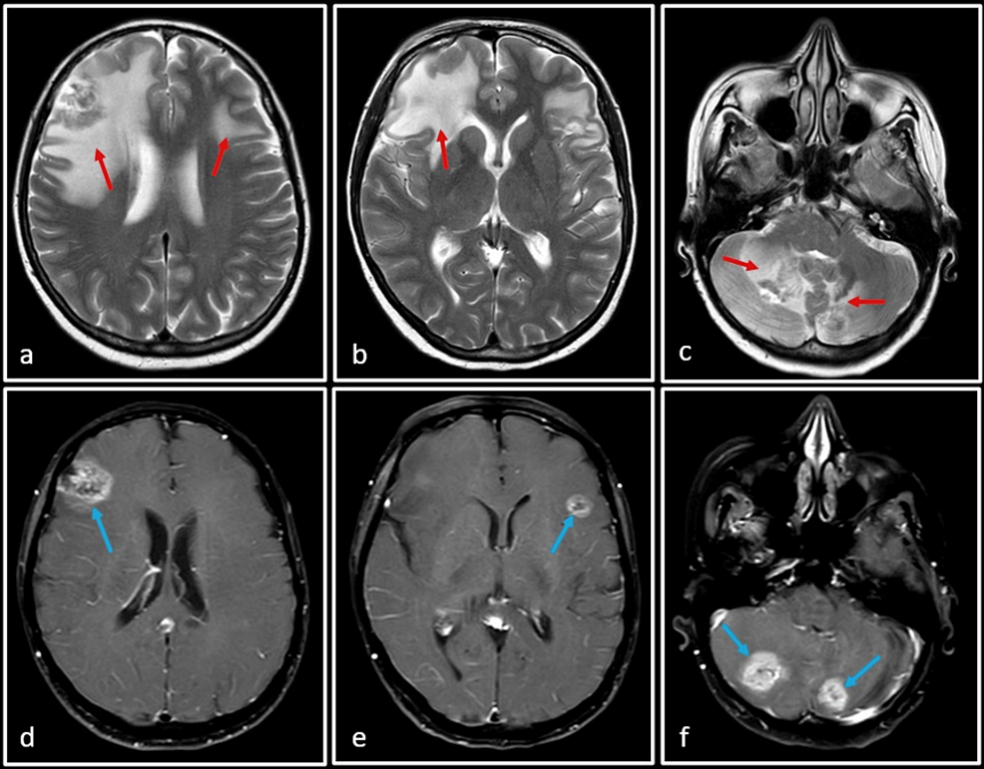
Follow-up MRI after three months of SRS to the frontal and cerebellar lobe lesions SRS: Stereotactic radiosurgery T2-weighted (a, b and c) and post-Gadolinium T1-weighted (d, e and f) images. There is an increase in size and enhancement of previously seen lesions (blue arrows) with an increase in the associated perilesional edema (red arrows). A working diagnosis of pseudoprogression was considered at this point of time and a follow-up MRI was requested.
